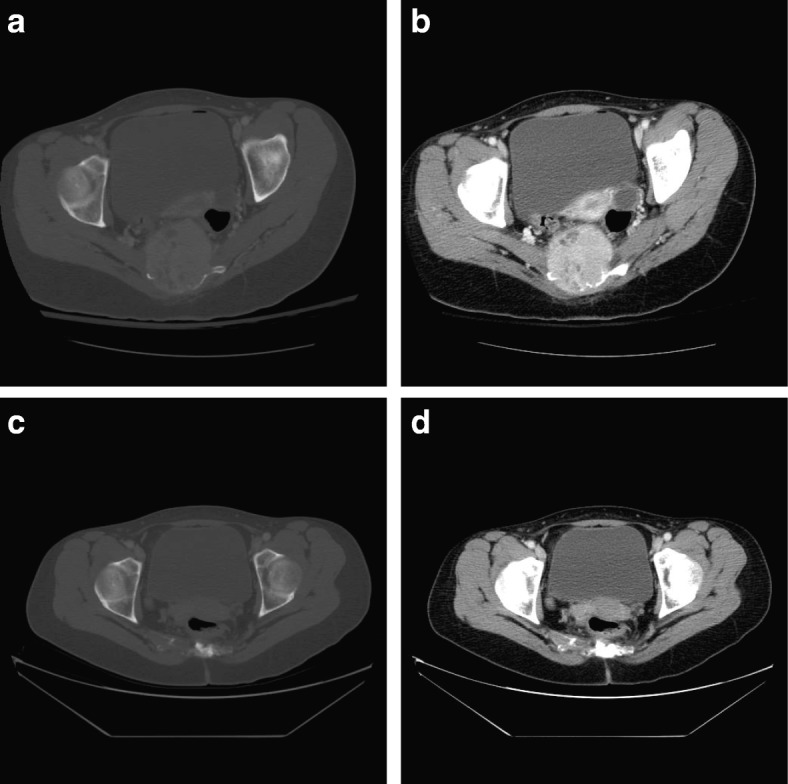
Sacral GCTB before and after treatment with denosumab. a Bone window and b soft tissue window pretreatment CT scan from August 14, 2009, through the level of the upper hip joints. There is extensive bone destruction and a large soft tissue mass that displaces the rectum. c Bone window and d soft tissue window CT repeat scan on December 12, 2013 (about 4 years and 4 months later) following treatment with denosumab (about 3 years and 10 months; first dose on January 21, 2010, and last dose on November 21, 2013). The soft tissue mass is now negligible, and the bone is reconstituting. *CT* computed tomography; *GCTB* giant cell tumor of bone
